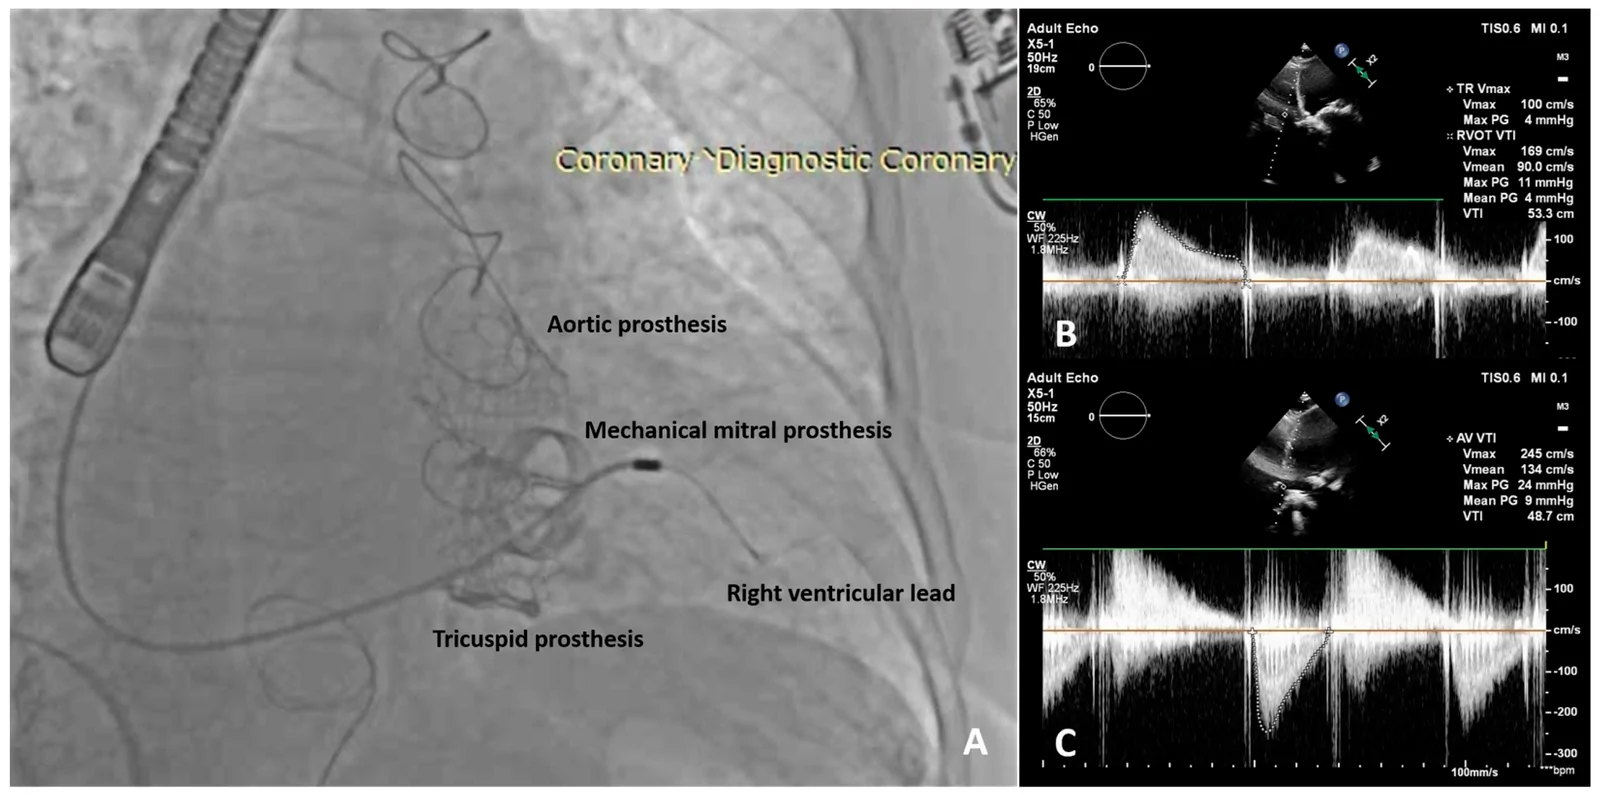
Final procedural and echocardiographic results. (A) Fluoroscopic image showing the three prosthetic valves (aortic, mechanical mitral, and tricuspid) together with the permanent right ventricular pacing lead. (B) Pre-discharge transthoracic echocardiography, apical four-chamber view, with continuous-wave Doppler across the tricuspid valve-in-valve prosthesis, showing a markedly reduced mean gradient of 4 mmHg and trivial residual regurgitation compared with the severe pre-procedural stenosis in Figure 1A. (C) Pre-discharge transthoracic echocardiography, apical five-chamber view, with continuous-wave Doppler across the transcatheter aortic valve, showing a mean gradient of 9 mmHg compared with the critical pre-procedural stenosis in Figure 1B. * bpm = beats per minute.
